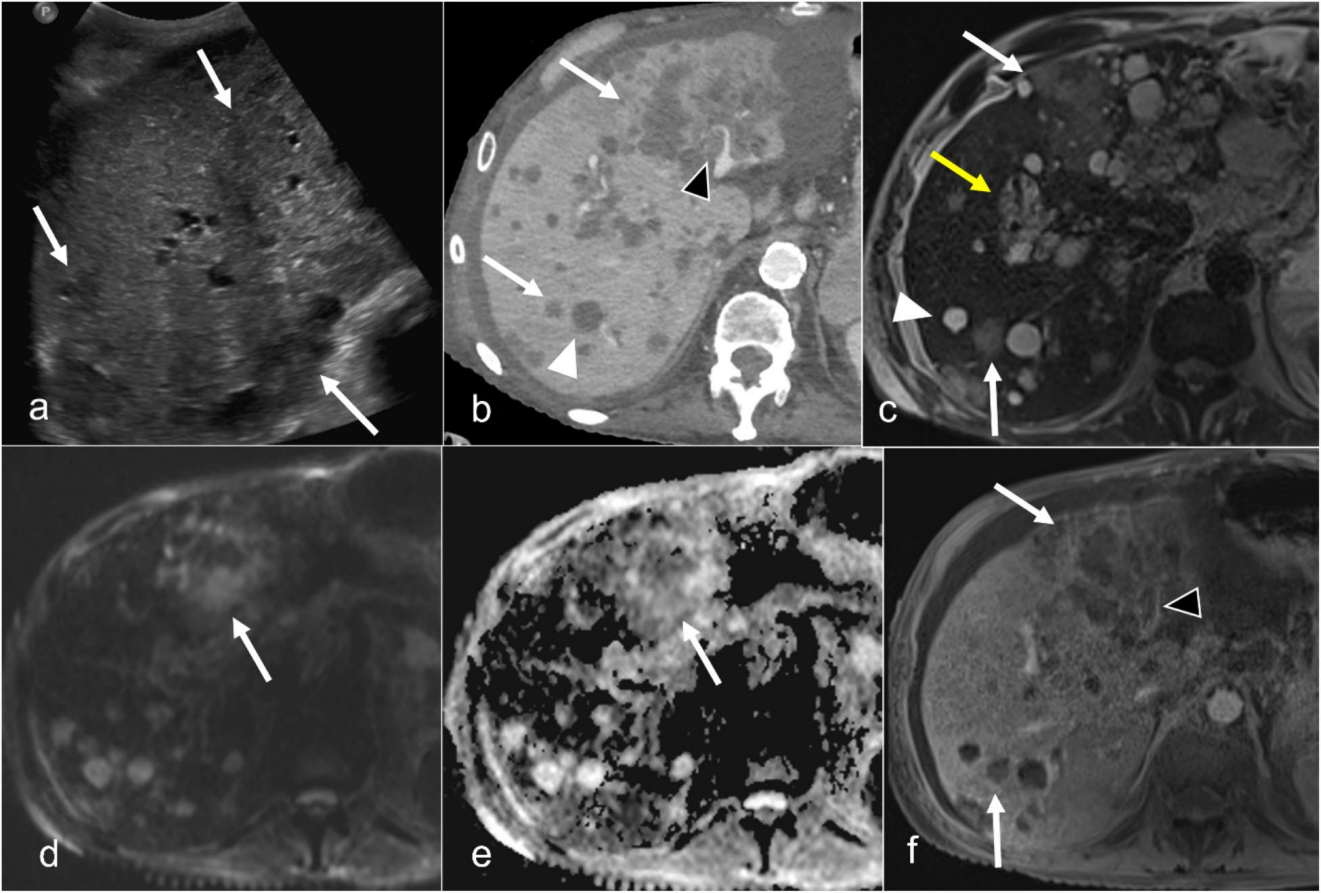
Intrahepatic abscess in a 72-year-old male patient with a history of adult polycystic kidney disease (ADPKD) and end-stage renal disease undergoing radiation treatment for esophageal cancer presents with gram-negative sepsis. Grayscale ultrasound image (a) demonstrates multiple areas of low echogenicity (arrows). Axial CT with contrast (b) shows mix of simple-appearing cysts (white arrowhead) and low-density lesions with subtle peripheral rim enhancement (arrows). Thrombus is present in the left portal vein (black arrowhead). MRI T2-weighted fat-saturated image (c) shows multiple simple cysts (arrowhead) secondary to ADPKD and T2-weighted hypointense lesions (arrows). Additionally, peribiliary cysts are seen (yellow arrow). Diffusion-weighted imaging (d) and ADC (e) images demonstrate signal restriction on the ADC map (arrow). Postcontrast T1-weighted image (f) shows lesions with peripheral rim enhancement and poor enhancement centrally. The left lobe lesion appears ill-defined because of the coalescence of multiple small lesions
